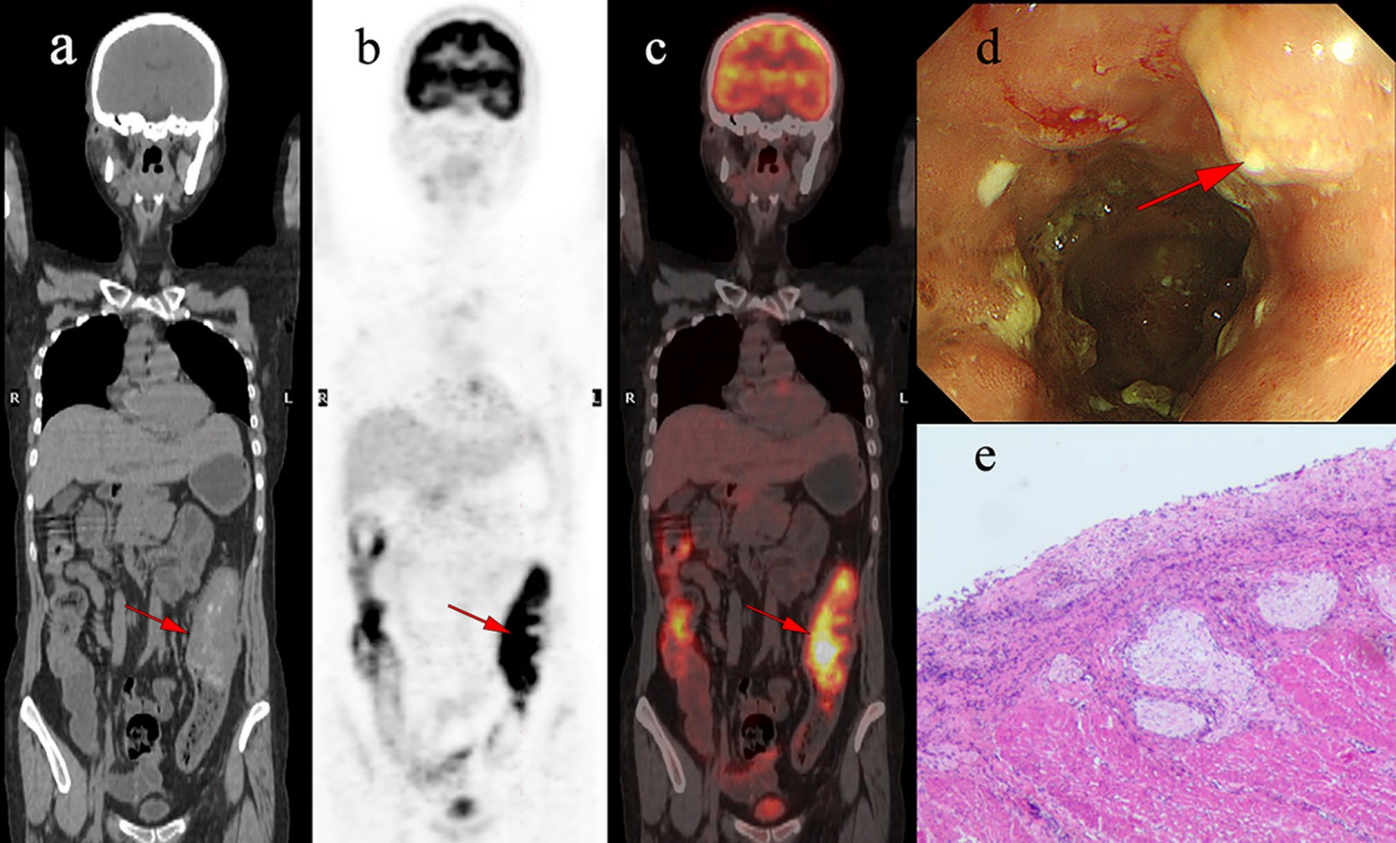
A 45-year-old woman underwent ^18^F-FDG PET/CT for unexplained abdominal pain. Incidental diffuse ^18^F-FDG uptake was found in the colon. The patient underwent colonoscopy and pathology examination. a-c Abnormal ^18^F-FDG PET/CT uptake in the colon. d shows diffuse thickening of the colon wall. e shows the pathological pattern of Crohn’s disease.
